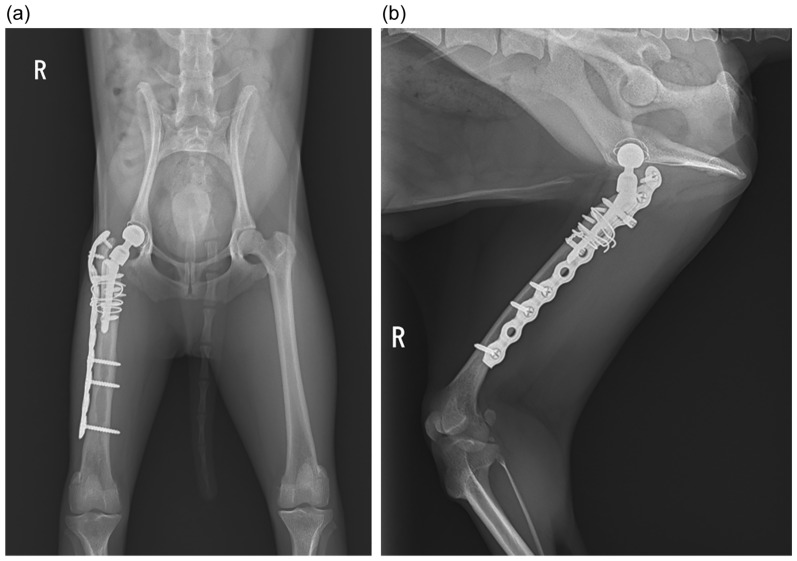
Postoperative radiographs 2 months after reimplantation: (a) ventrodorsal view; (b) yoga view (Cr-Cd view tangent to the stem).
